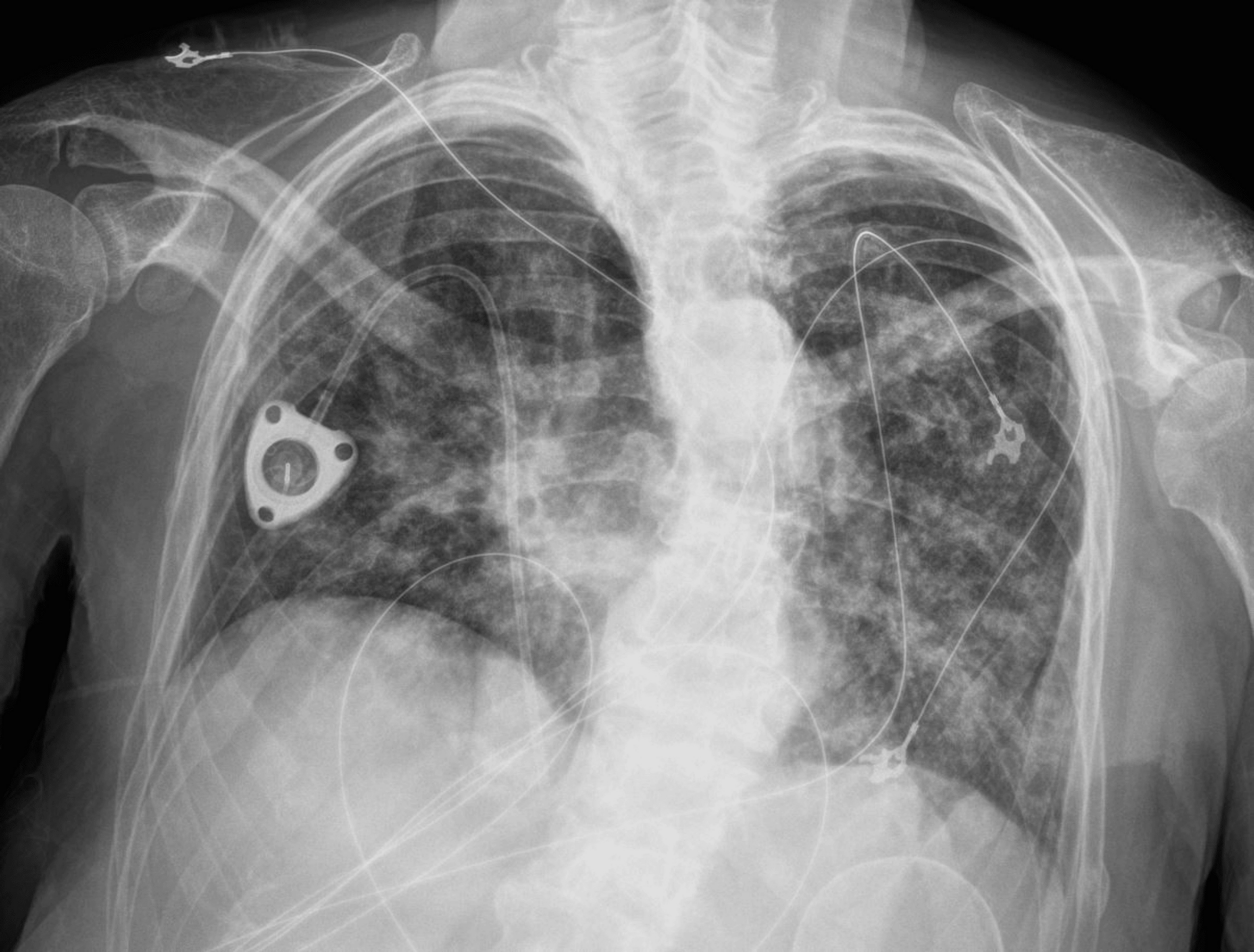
Chest radiography at admission
